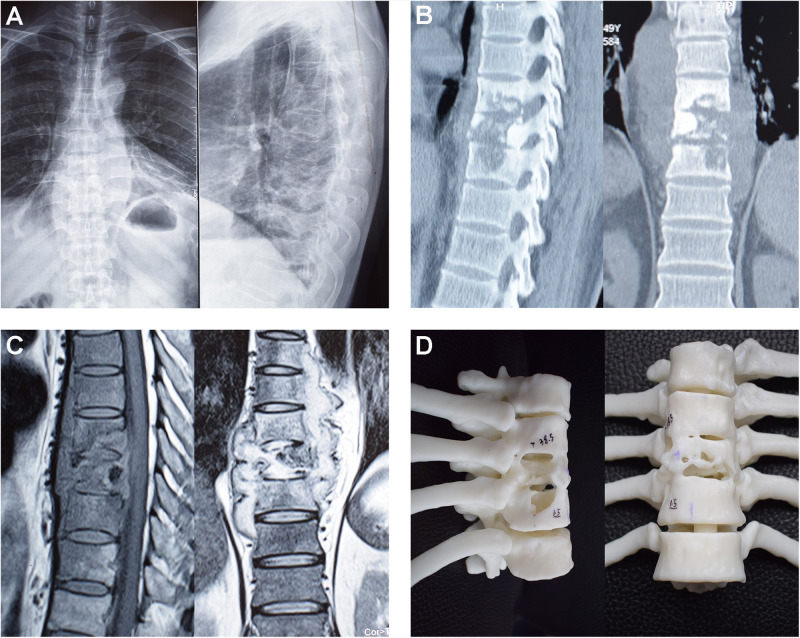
Preoperative imaging results of the patient with thoracic spinal tuberculosis. (A) preoperative anteroposterior and lateral radiography; (B) preoperative CT images; (C) preoperative MRI; (D) preoperative 3D printed model.
